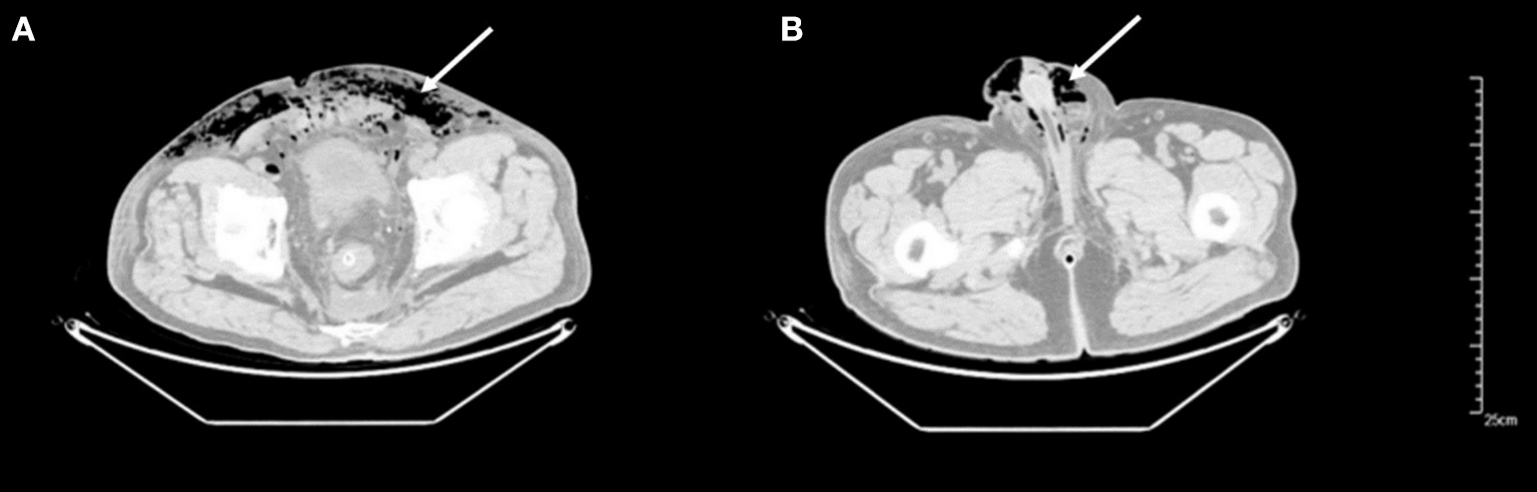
CT imaging (axial views) demonstrating subcutaneous gas of lower abdomen (A) and scrotum (B) indicated by white arrows.
